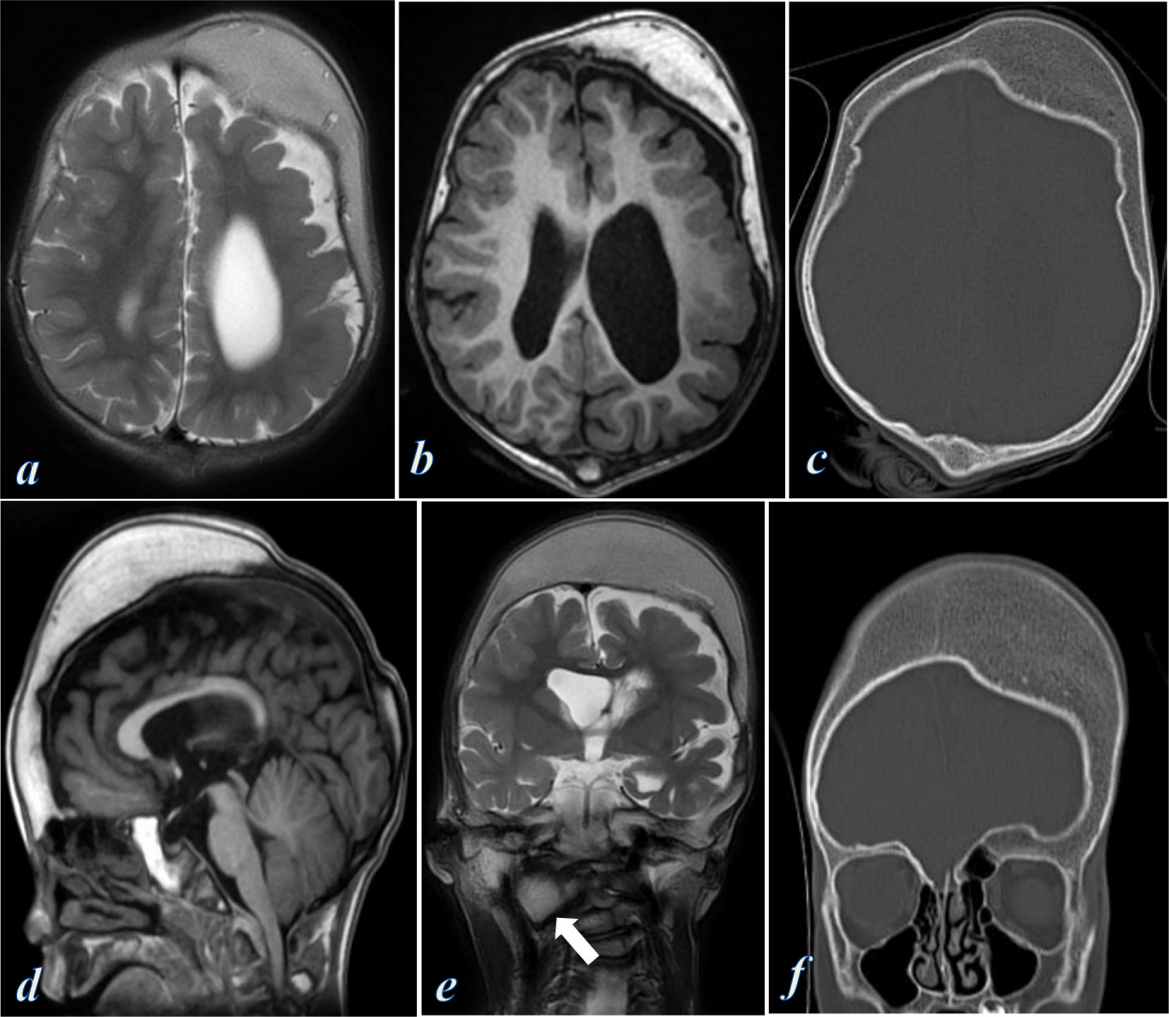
MRI and CT examination of the brain; (a) axial T_2_ weighted image shows asymmetric left frontoparietal calvarial thickening eliciting high signal, with dysplastic changes of the left cerebral hemisphere showing thick cortex, cortical pachygyria, abnormal grey–white matter differentiation, thin white matter, and large left lateral ventricle. (b) Axial *T*
